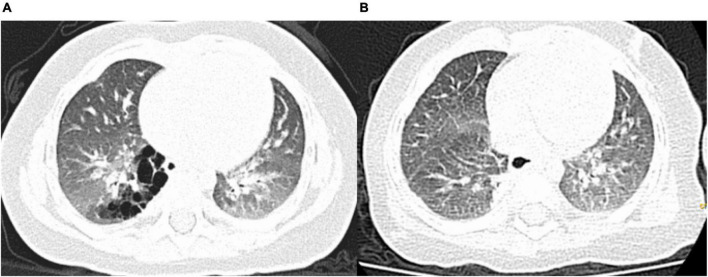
Pre- and postoperative computed tomography (CT) findings. (A) Preoperative CT showing a congenital pulmonary airway malformation in the right S10; (B) Follow-up CT scan obtained 3 months after the operation showing no residual lesions.
